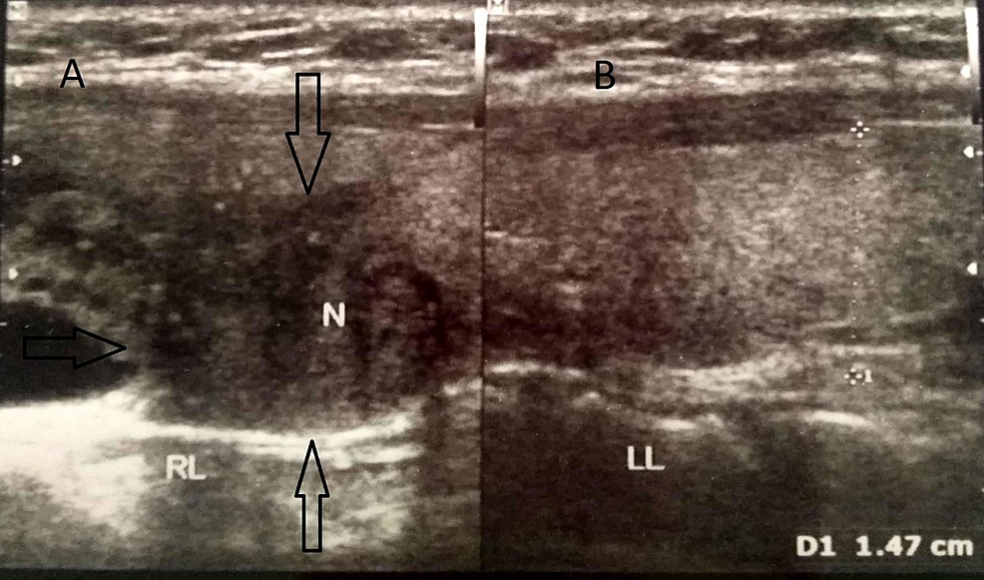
(A) USG of the thyroid and parathyroid glands showing (arrows) an irregular hypoechoic nodular lesion measuring approximately 17 × 14 mm having foci of calcification in the upper pole of the right lobe of the thyroid gland suggestive of adenoma or carcinoma of the parathyroid gland. (B) No abnormalities were found on the left side. USG: ultrasonography
